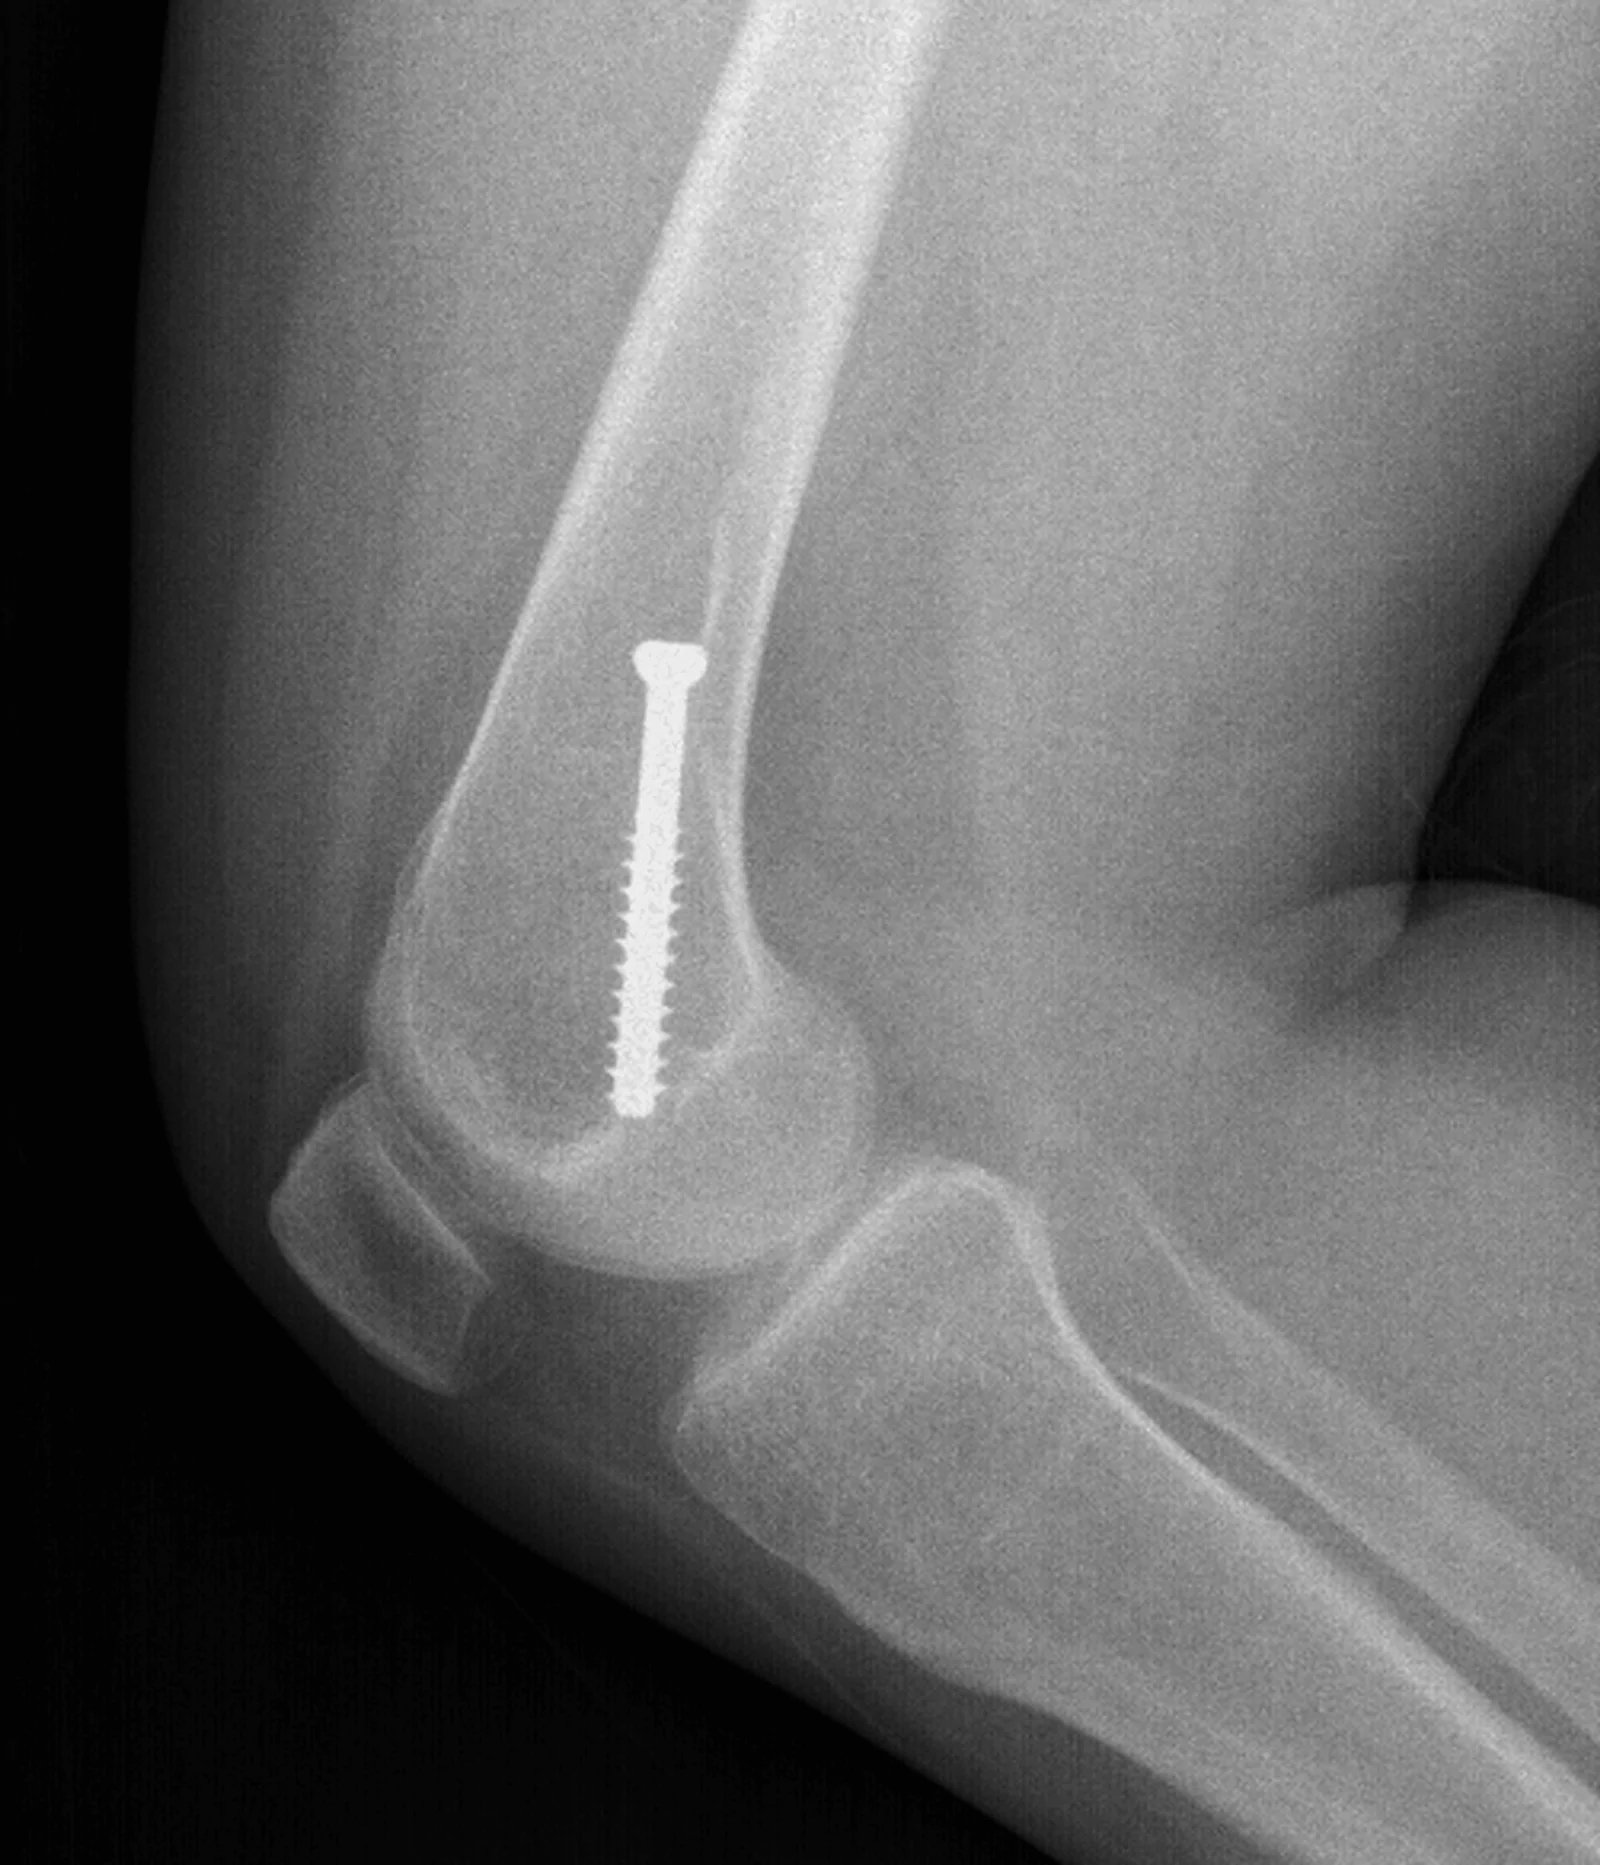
Lateral radiograph of the right knee showing medial distal femoral epiphysiodesis performed to correct angular deformity secondary to longstanding rickets.
